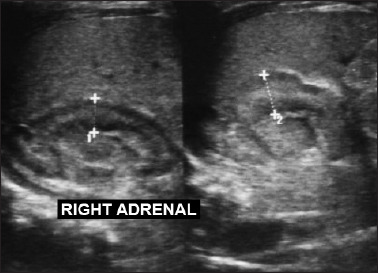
Abdominal ultrasonography showing blood collection around the right adrenal gland, which was enlarged and heterogeneous, consistent with right adrenal hemorrhage.
